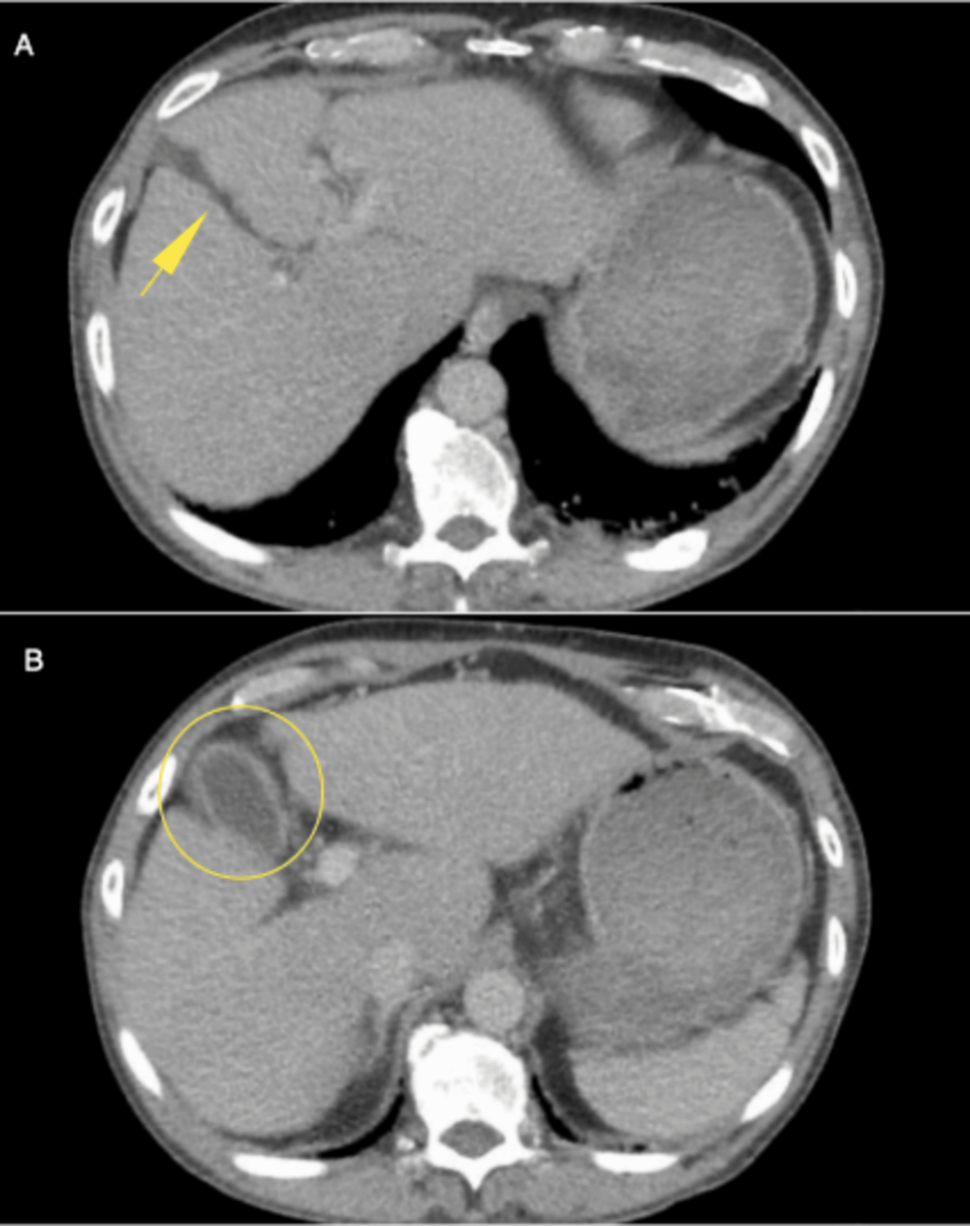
A) Contrast-enhanced CT showing grade III hepatic injury in segment V (yellow arrow); B) Contrast-enhanced CT showing mild gallbladder wall thickening (transparent yellow circle). CT, computed tomography
